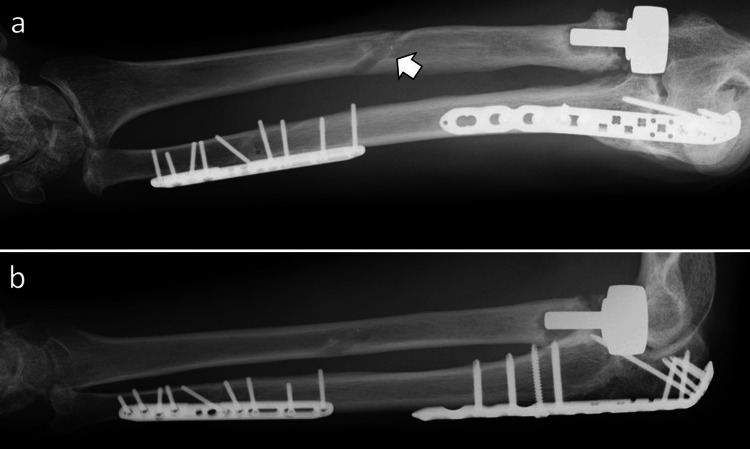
X-ray images at the final observation. (a) Anteroposterior and (b) lateral plain radiographs of the left forearm. Both the fracture and osteotomy site are united. An oblique bone tunnel can be seen in the shaft of the radius (white arrow).
